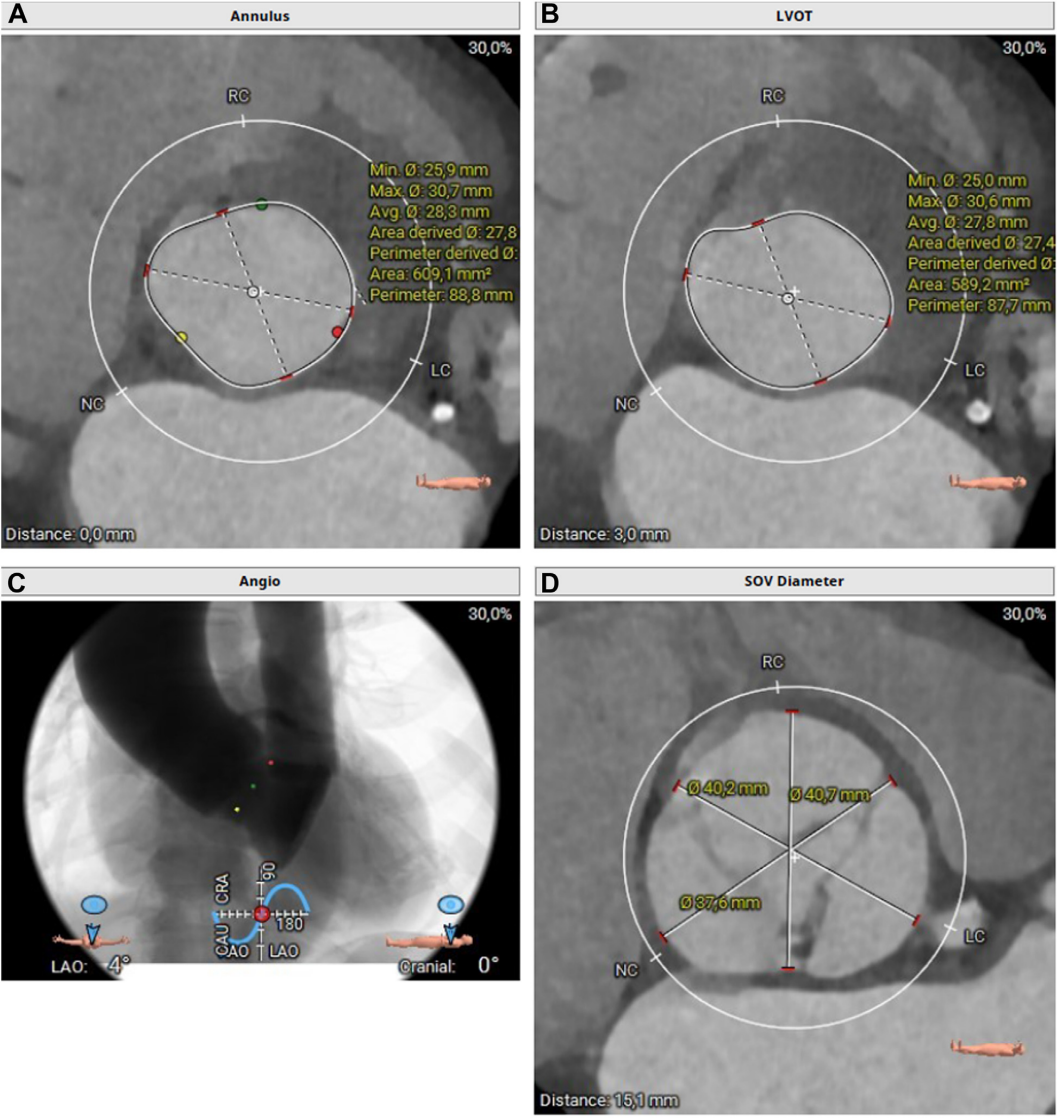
Annular, LVOT, and SOV Measurements on Preoperative CT (A to C) Index CTA images with annular, LVOT, and SOV measurements. (D) CT images of working projections. CT = computed tomography; CTA = computed tomography angiography; LAO = left anterior oblique; LCC = left coronary cusp; LVOT = left ventricular outflow tract; NCC = noncoronary cusp; RCC = right coronary cusp; SOV = sinus of Valsalva.
